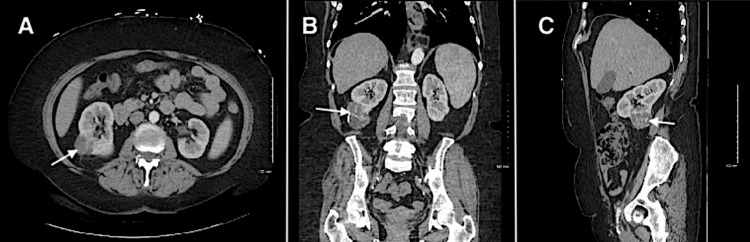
Computed tomography of the abdomen/pelvis demonstrating a large complex Bosniak IV mixed solid-cystic lesion in the right kidney (arrow) in axial (A), coronal (B), and sagittal (C) planes.
